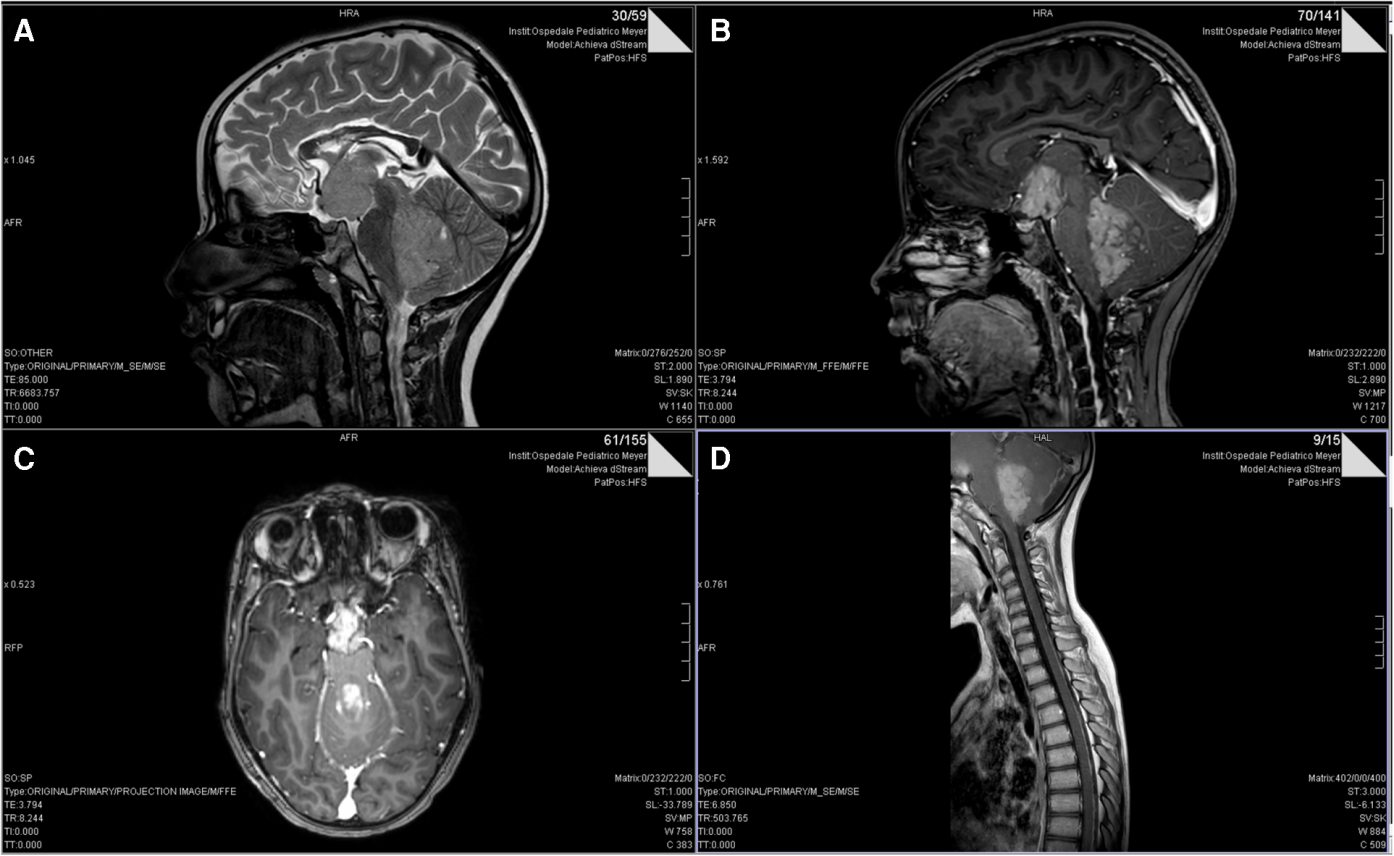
Pre-operative T2 (A) and post-gadolinum (B) sagittal and axial (C) MRI scans of our patient showing the extensive metastasis to hypothalamus. (D) post-gadolinium-enhanced T1-weighted sagittal spine MRI image showing leptomeningeal dissemination of medulloblastoma.
